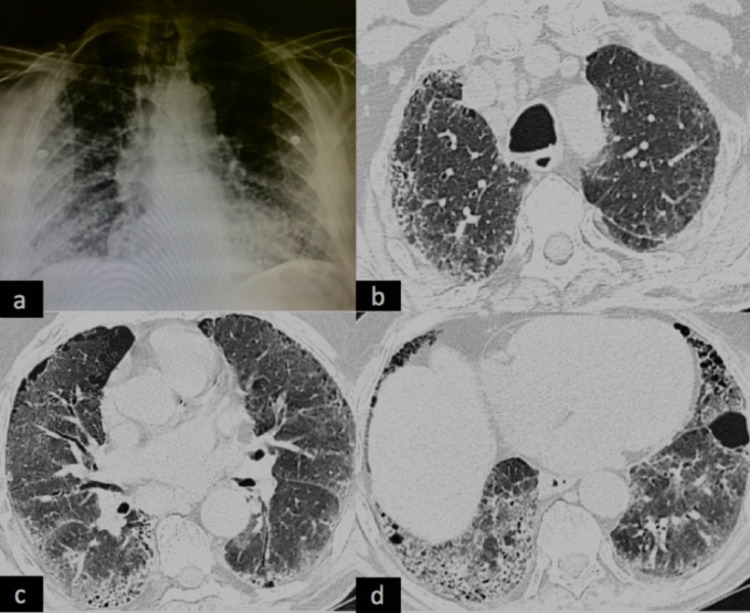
(a) Chest X-ray showing bilateral middle and lower zone noninhomogeneous opacities. (b-d) HRCT of the chest showing ground-glass opacities superimposed on UIP pattern. HRCT, high-resolution computed tomography; UIP, usual interstitial pneumonia
